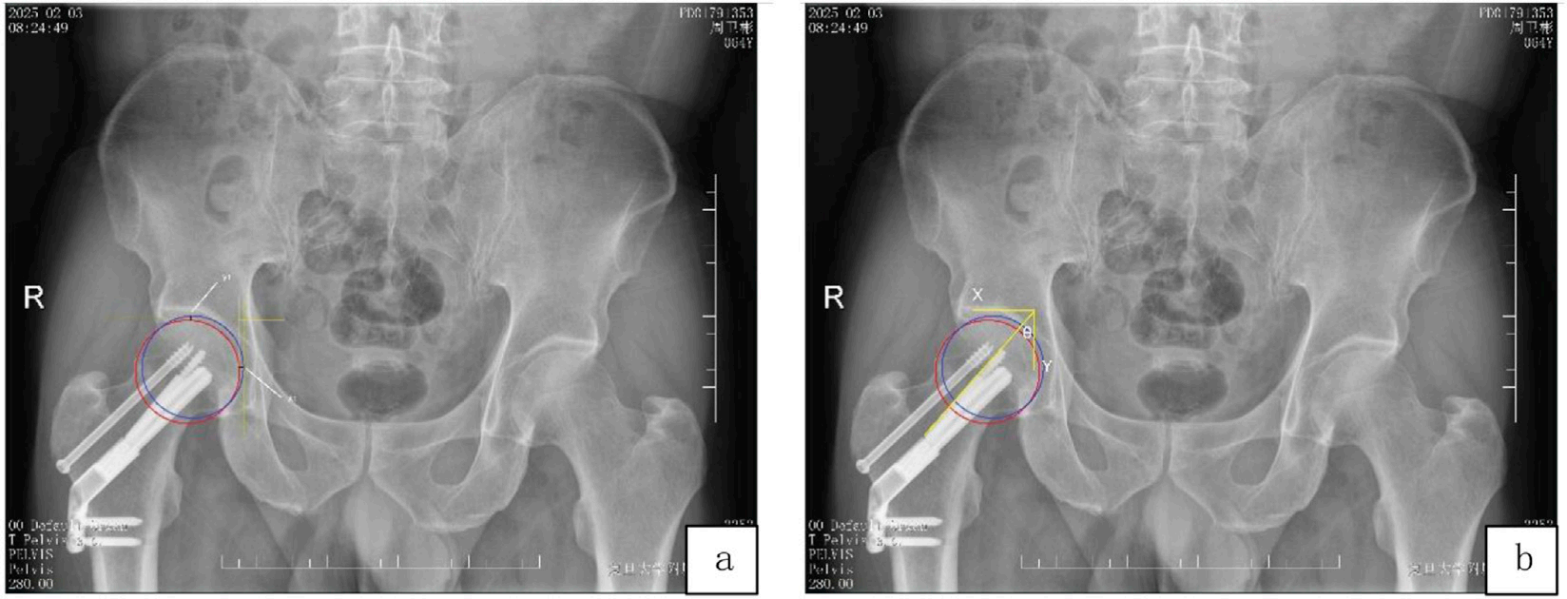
Measurement of femoral neck shortening length after FNS fixation for femoral neck fracture: (a) In the anteroposterior pelvic radiograph, superimpose the image of the greater trochanter of the healthy side with that of the affected side. Subsequently, draw a blue circle to represent the femoral head of the healthy side and a red circle for the femoral head of the affected side. The variables x1 and y1 denote the displacement lengths of the femoral head of the affected side relative to the femoral head of the healthy side along the horizontal axis (x-axis) and vertical axis (y-axis), respectively; (b) The angle θ represents the angle between the femoral head-neck axis and the vertical axis (y-axis). The femoral neck shortening length |z| (mm) is calculated as |y1 sinθ + x1 cosθ|.
